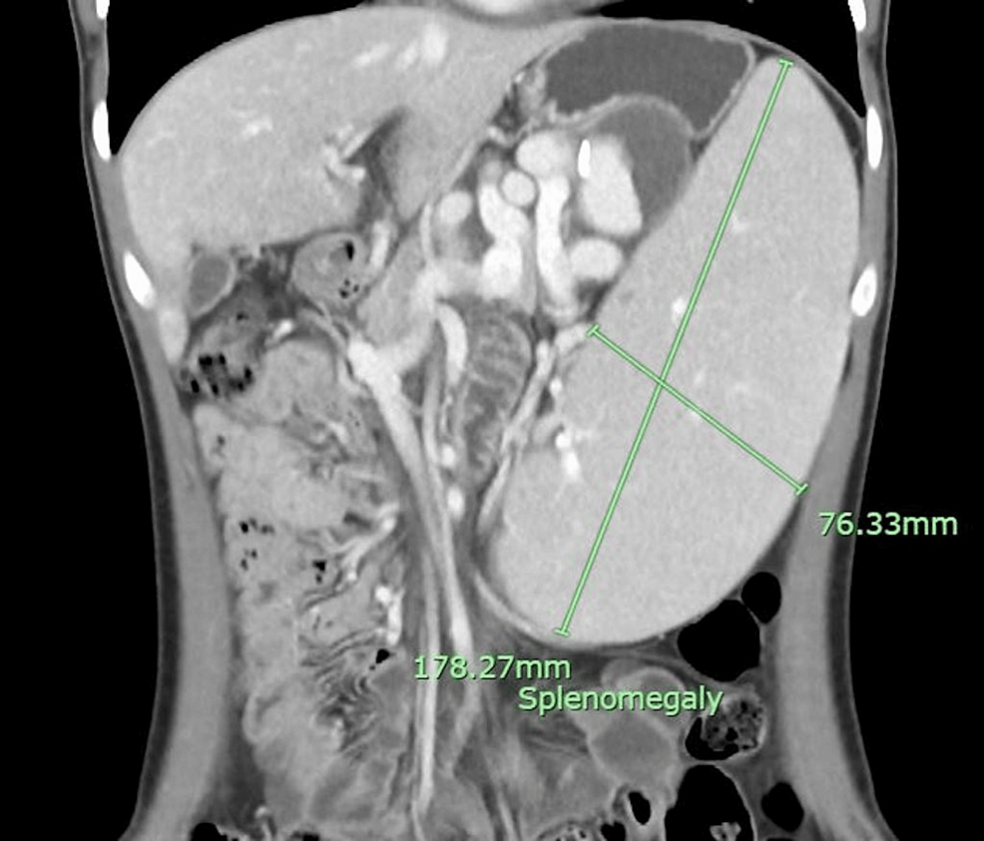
Computed tomography (CT) scan (coronal view) showing splenomegaly
